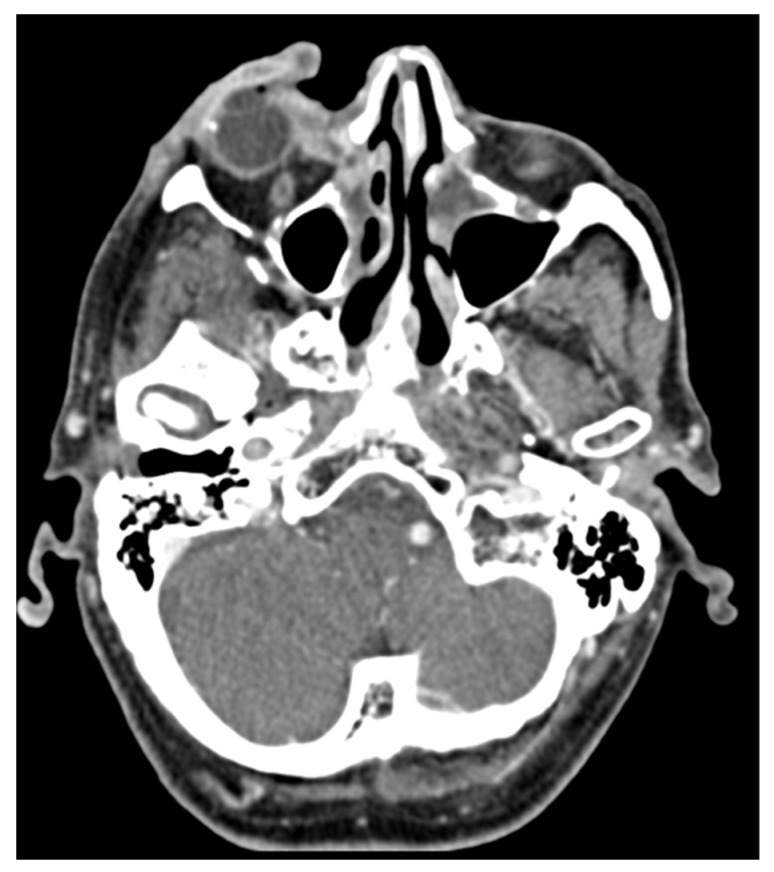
An 86-year-old male with squamous carcinoma of the right orbit, CT examination performed with administration of intravenous contrast shows the ulcerated skin and subcutaneous lesion in correspondence with the right orbit with peripheral enhancement with centrally necrotic-colliquative components. (Dual Source CT (Siemens Somatom), Siemens Healthcare, Forcheim, Germany).
